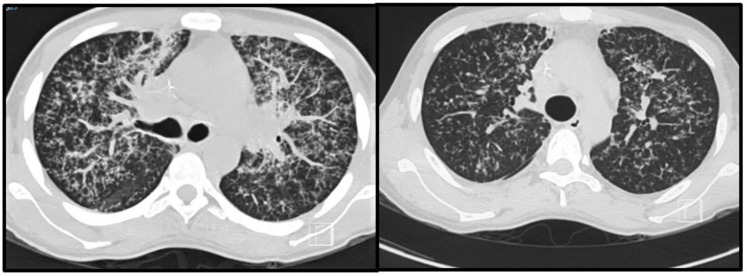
Pulmonary images of a 19-year-old male patient with subacute pulmonary coccidioidomycosis: axial thoracic CT showing bilateral peribronchovascular and perilymphatic nodules in both lungs.
